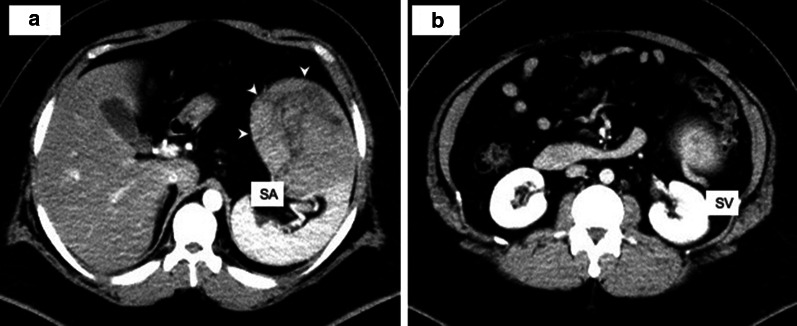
Enhanced computed tomography. Enhanced computed tomography showing a solid tumor (arrow) on the ventral side of the spleen with a clear border of approximately 14 cm continuous with the spleen. The inside mainly contained fat components around the blood vessels, while the solid part was imaged incrementally (a). Blood was supplied from the splenic artery (a), and dilated blood vessels, thought to be drainage veins, were found in the tumor to the caudal side, joining the splenic vein (b). *SA* splenic artery, *SV* splenic vein
